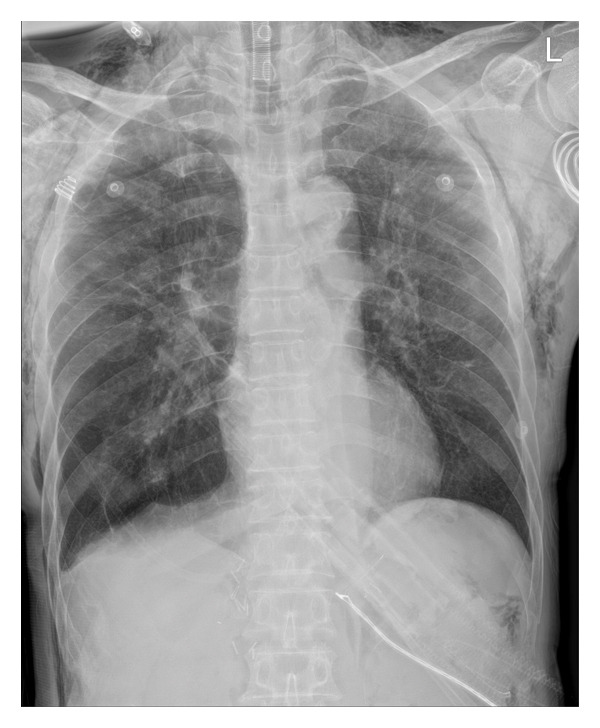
Portable chest radiograph obtained 1 h postoperatively showing spontaneous resolution of the pneumothorax. Lung expansion is restored, and the mediastinum has returned to the midline. The patient recovered without chest tube insertion.
